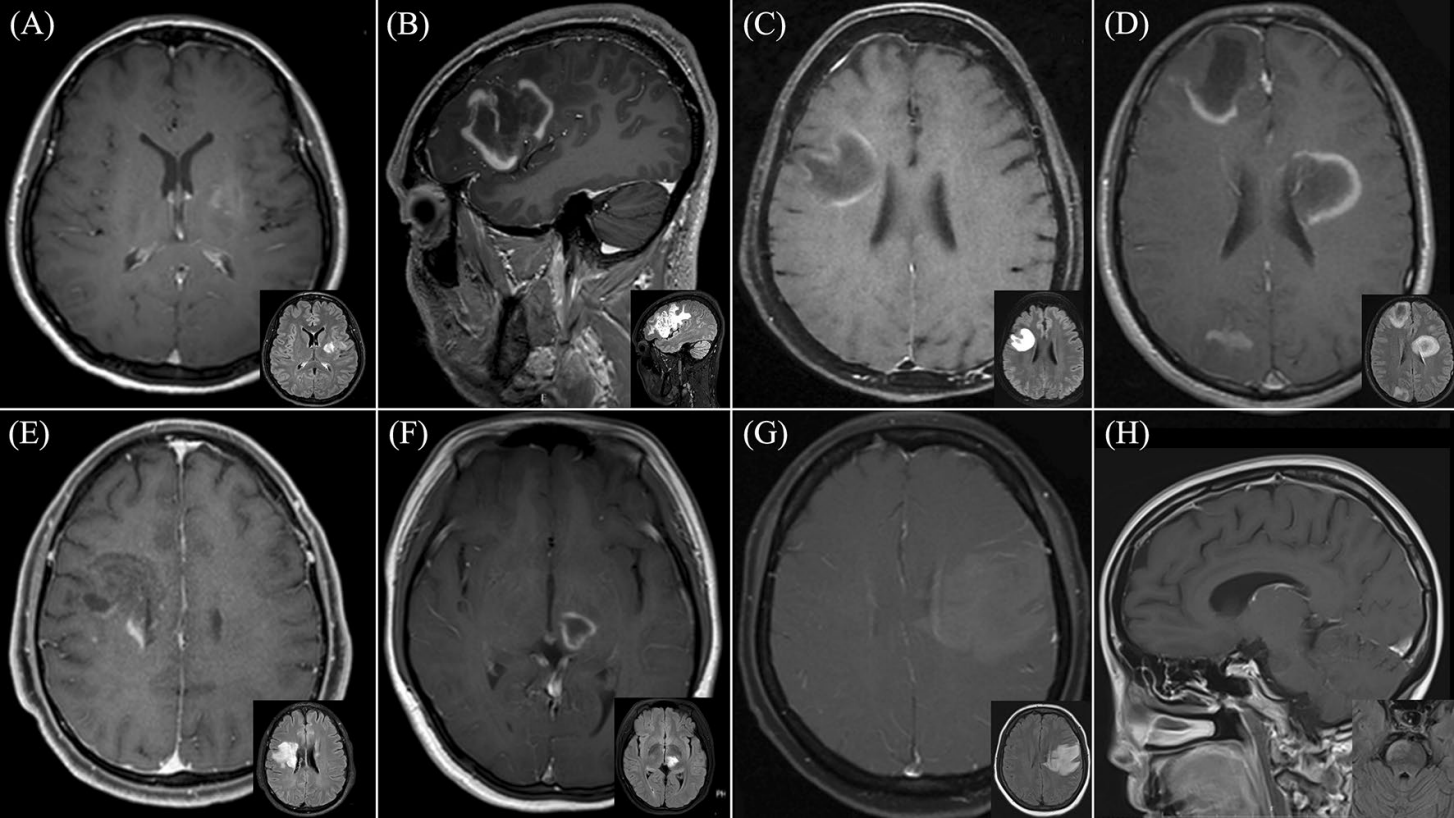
Representative brain magnetic resonance imaging (MRI) in six patients diagnosed with tumefactive demyelinating lesions (TDL). Post-gadolinium T1-weighted imaging is displayed in the larger picture, with a smaller Fluid-Attenuated Inversion Recovery (FLAIR) MRI provided in the lower right corner. (A) Patient 1: Heterogeneous enhancing pattern of the left basal ganglia lesion. (B) Patient 2: Open ring pattern of the left frontotemporal lesion. (C) Patient 12: Open ring pattern of the right frontoparietal lesion. (D) Patient 17: Open ring pattern of three lesions at the right frontal, left frontoparietal, right parietooccipital region. (E) Patient 20: Heterogeneous enhancing pattern of the right frontal lesion with Balo-like appearance. (F) Patient 22: Open ring pattern of the left thalamic lesion. (G) Patient 23: Patchy enhancing pattern of the left frontoparietal lesion. (H) Patient 25: Open ring pattern of the right-sided pontine lesion.
